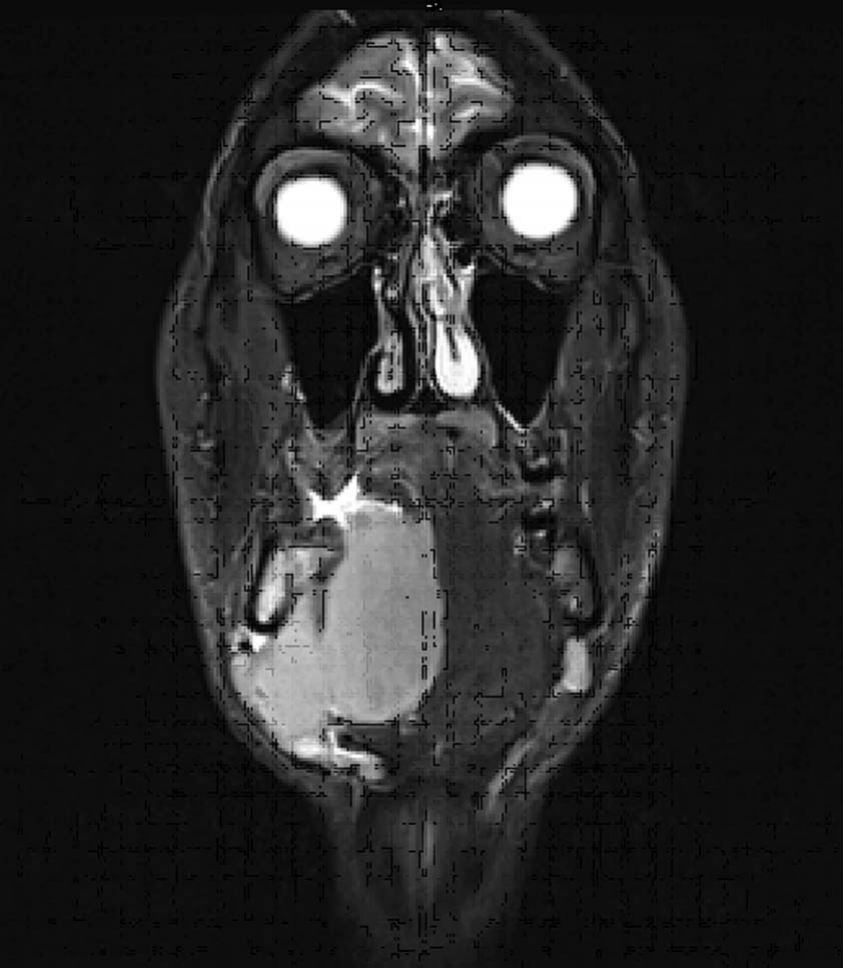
Coronal MRI scan revealing a bone destroying mass of approximately 7 × 8 × 6 cm^3 ^surrounding the mandible and massively infiltrating the soft tissue of the floor of the mouth and the tongue.
